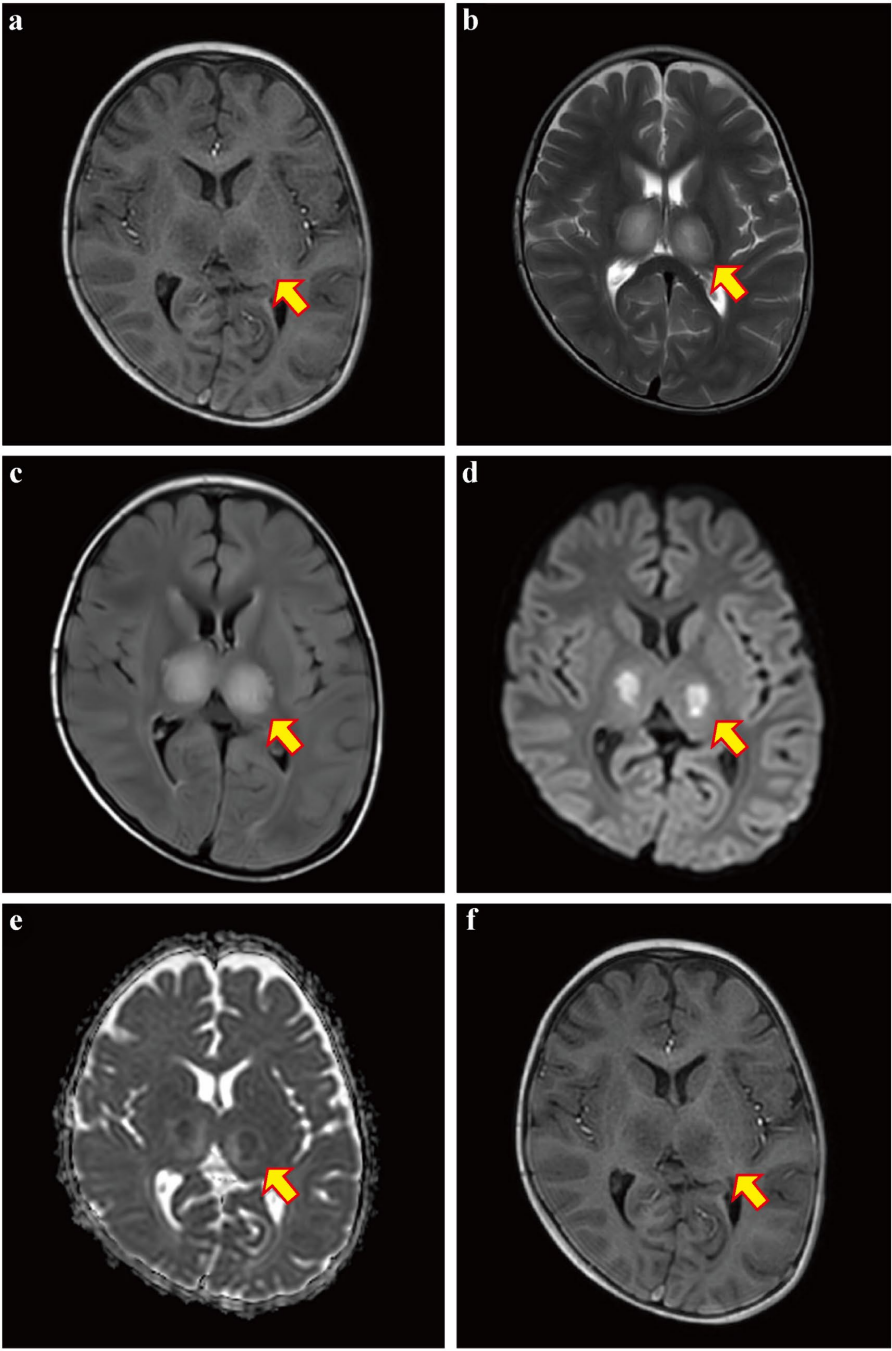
MRI images of a child with Omicron BA.5 infection, showing damage to the thalamus. a T1WI showed a low signal; b T2WI showed a high signal; c FLAIR water suppression showed a significantly high signal; d DWI showed a significantly high signal; e ADC showed a slightly high signal at the lesion's periphery and a low signal at the center; f DWI showed a slightly increased signal in the splenium of the superior corpus callosum. *MRI* magnetic resonance imaging, *FLAIR* fluid attenuated inversion recovery*, ADC* apparent diffusion coefficient*, DWI* diffusion weight imaging
